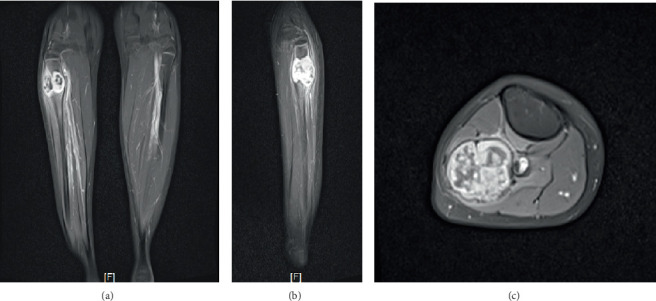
Magnetic resonance imaging. (a) Coronal view. (b) Sagittal view. (c) Axial view.
